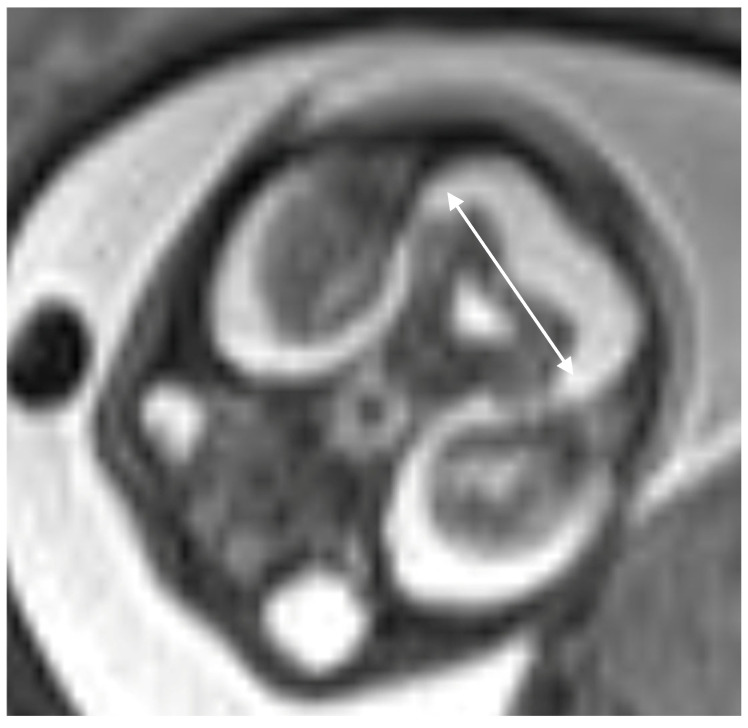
Fetal MRI axial T2W sequence at 21st week of gestation shows small cerebellum. Transcerebellar diameter is smaller than −3 SD (white double arrow).
